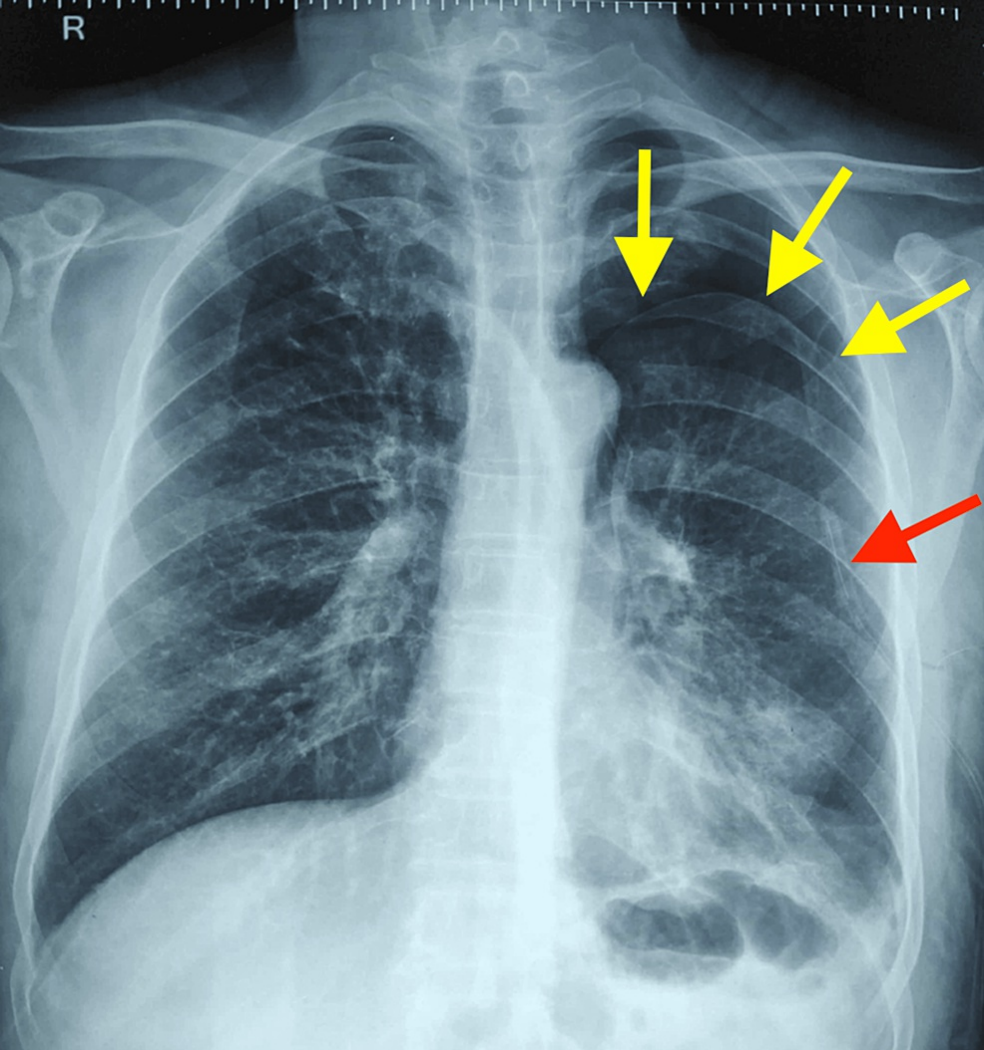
Chest X-ray showed left-sided pneumothorax (yellow arrows) and ICT in situ (red arrow).
